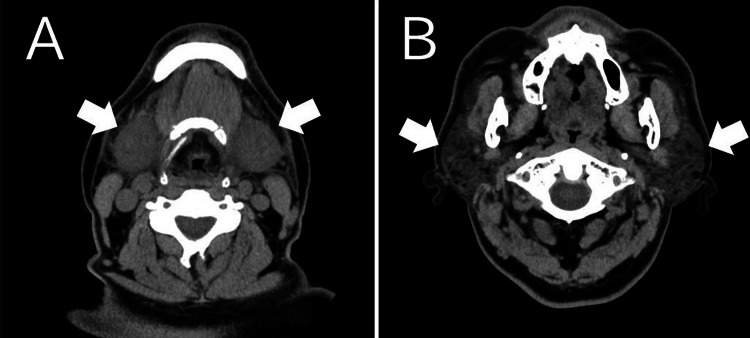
CT findings (HOD1). (A) Arrows indicate swelling of the bilateral submandibular glands (50 × 38 mm). (B) Arrows indicate swelling of the bilateral parotid glands (34 × 26 mm) CT: computed tomography, HOD1: hospital day 1
